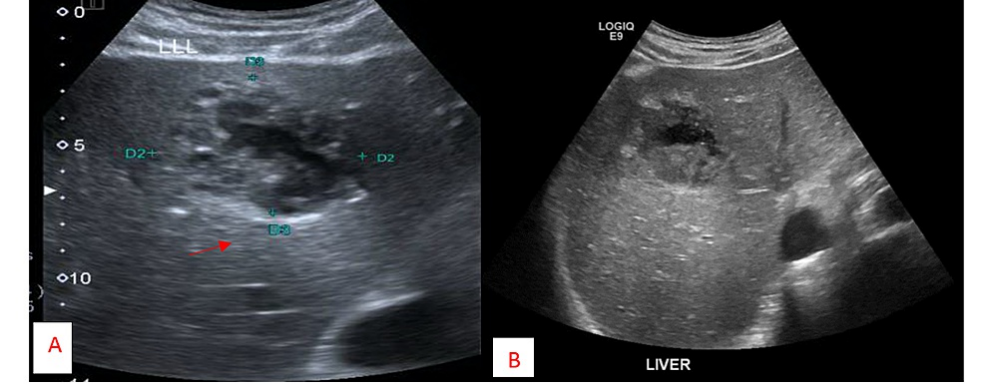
Ultrasound of the abdomen A. Ultrasound of the abdomen showing abscess of dimensions 6.3 x 6 x 5.1 cm and estimated volume of 94 cc. B. Repeat ultrasound performed 15 days after ultrasound-guided abscess drain showing regression in the liver abscess of dimensions 4.4 x 4.8 x 4 cm and estimated volume of 54 cc
